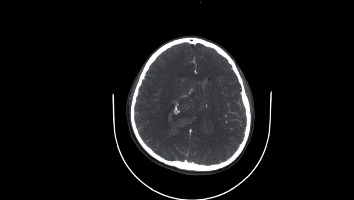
Head CT axial view with contrast.
